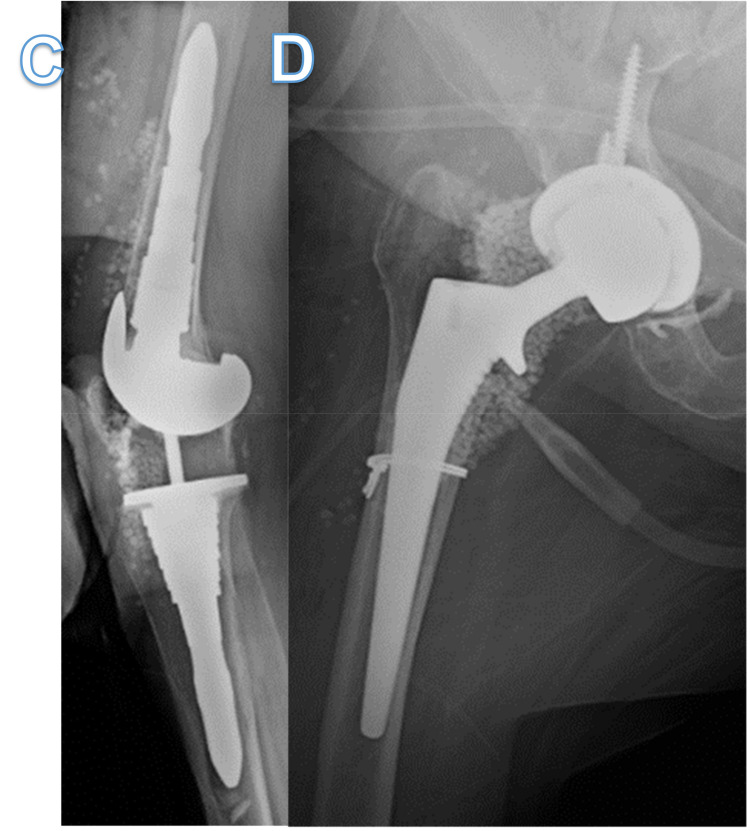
X-ray of knee and hip before and after bacteriophage therapy. (A,B) X-ray anterior-posterior view of right knee and hip showing temporary antibiotic-coated knee prosthetic and Prostalac hip spacer with residual cerclage wire and screw. (C,D) X-ray lateral view knee and anterior-posterior view hip showing total knee and hip arthroplasties implanted after bacteriophage therapy and subsequent proven sterility of the joints. Retained cerclage wire still present.
